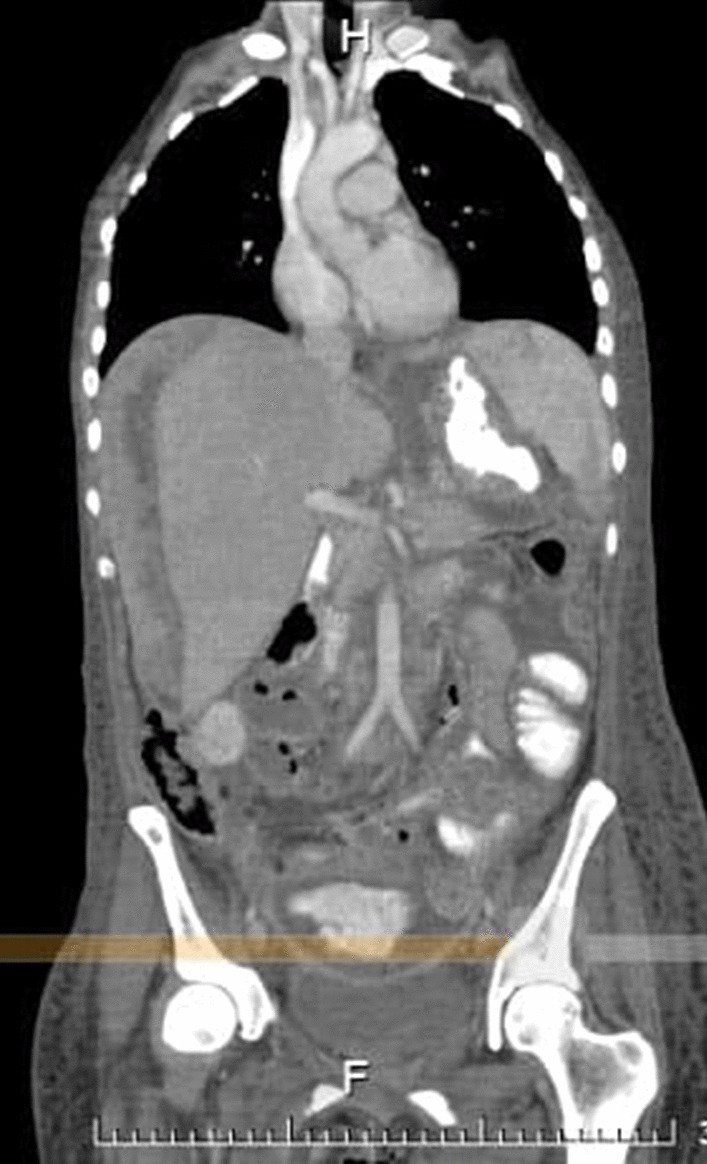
Contrast-enhanced computed tomography of the thorax, abdomen, and pelvis. Postsurgical findings. Image showing bilateral pleural effusion with passive atelectasis of adjacent segments in the left lung, absent uterus due to surgical intervention, and the presence of hydro pneumoperitoneum with air bubbles in the right subphrenic space. An engrossment of the gastric fundus is apparent
